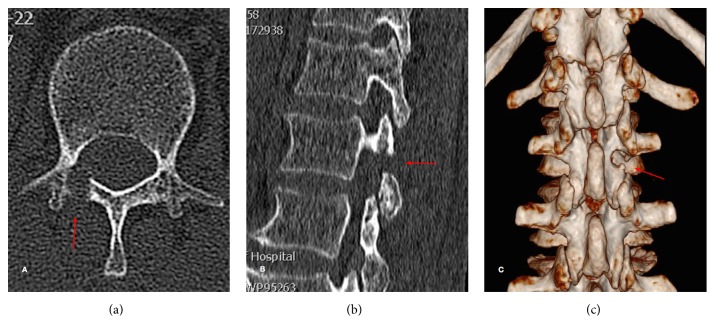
Postoperative computed tomography (CT) scans. The bone resection of the lamina is clearly indicated by CT scans in the axial (a) and sagittal (b) views. Reconstructed CT scans demonstrating no injury on the articular process and the pedicle of the vertebral arch (c).
